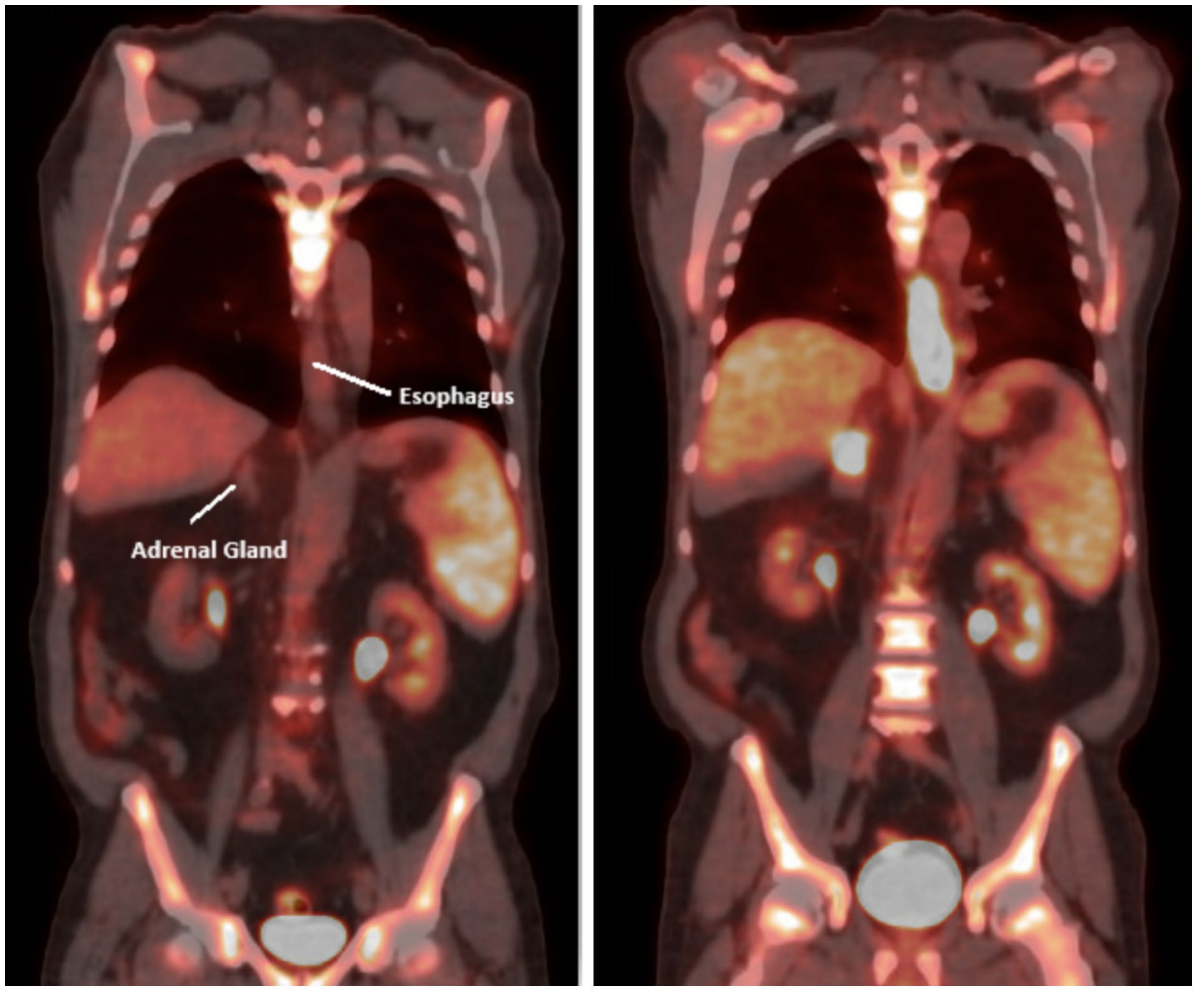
PET scan coronal view after 4–5 cycles of chemotherapy (left) showing interval decrease in the circumferential thickening and FDG activity associated with the distal 3rd of the thoracic esophagus and repeat PET scan after 2 additional cycles showed significant progression of disease both in the esophagus and adrenals (right).
